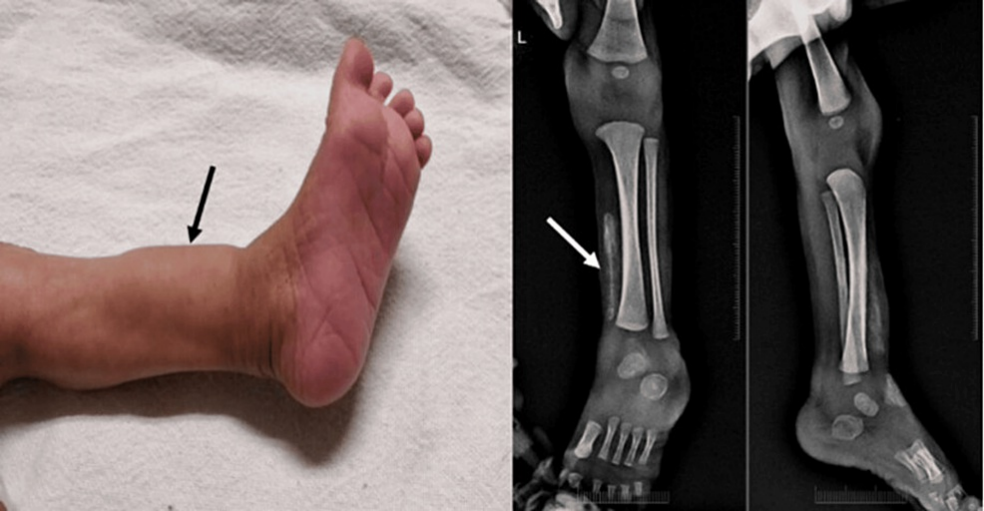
(a) Firm, indurated swelling over the dorsal aspect of the left leg in proximity to the previous cannulation site. (b) Anterior-posterior and lateral view radiographs depicting soft tissue calcifications in the left lower limb
